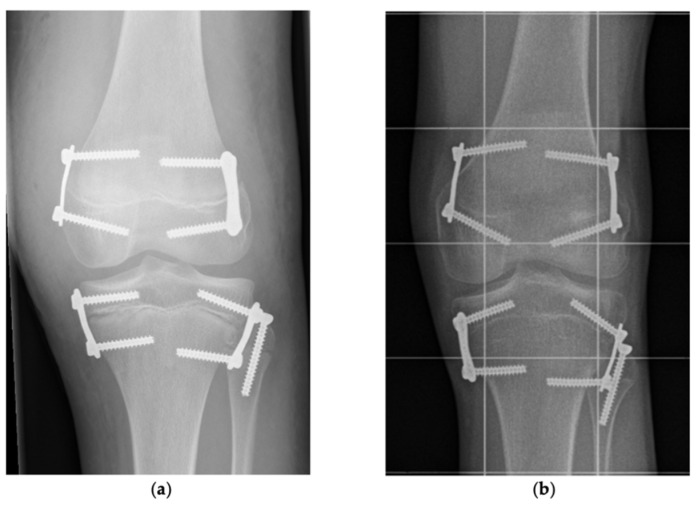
Proximal tibial epiphysiodesis with proximal fibular epiphysiodesis. (a) After implantation: Anteroposterior radiograph of a left knee showing proximal tibial epiphysiodesis with PediPlates^TM^ and proximal fibular epiphysiodesis with 36 mm fully threaded cannulated screw in 15-year-old boy with lateral collateral ligament (LLD) of 3.3 cm. (b) Before implant removal: Same patient after a treatment time of 36.5 month. The fibular head is localized more distal than the center of the proximal tibial growth plate indicating fibula shortening (dPTFH = −14.5 mm). dPTFH = Distance between the center of the proximal tibial growth plate and a line tangential to the tip of the fibular head horizontal to the imaging plane.
